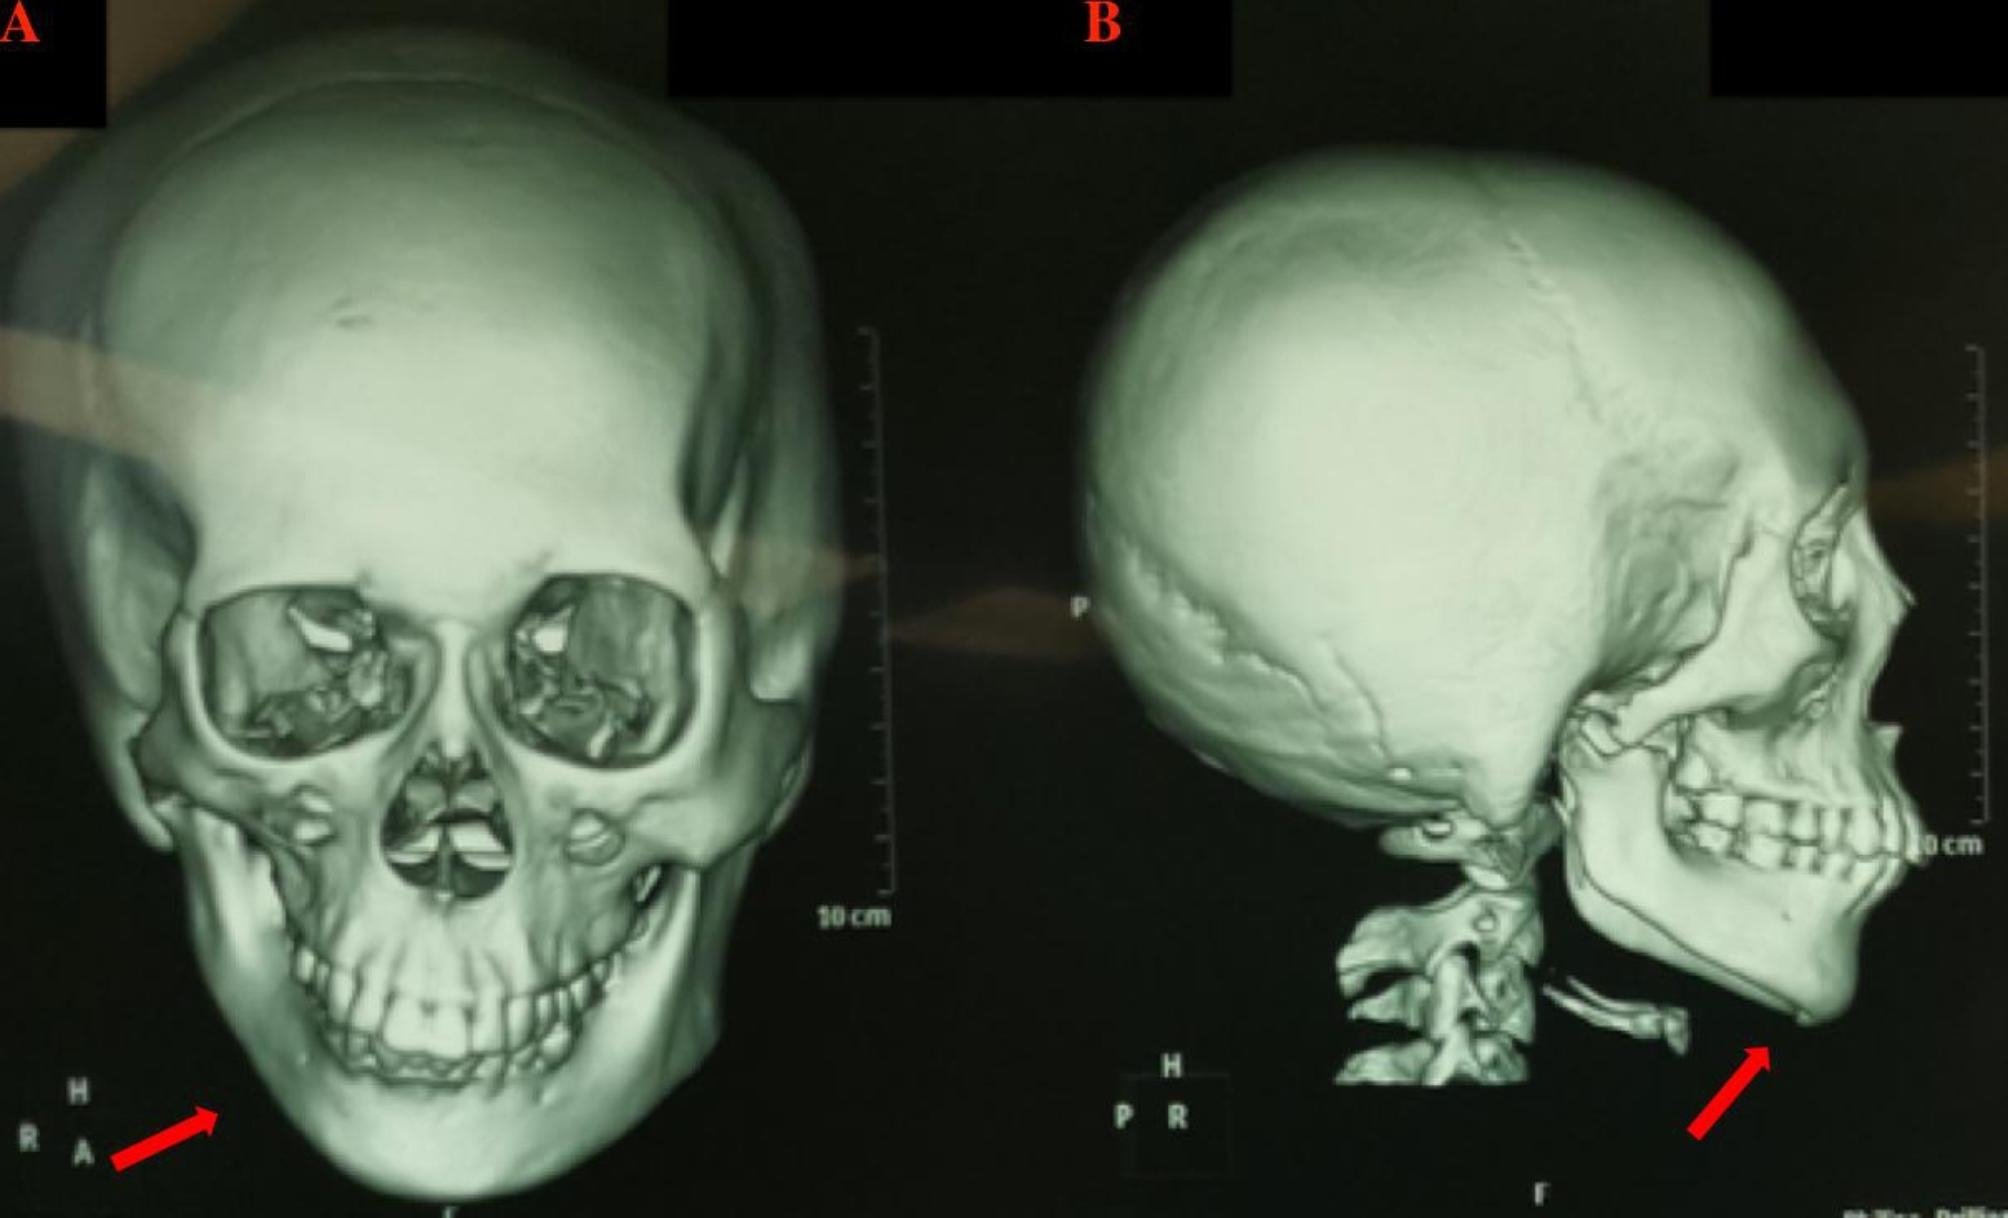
A: The cranial CT imaging of the patient shows that the right mandibular is shorter than the left. The arrow shows that right sided mandible hypoplasia. B: Right side of this patient
